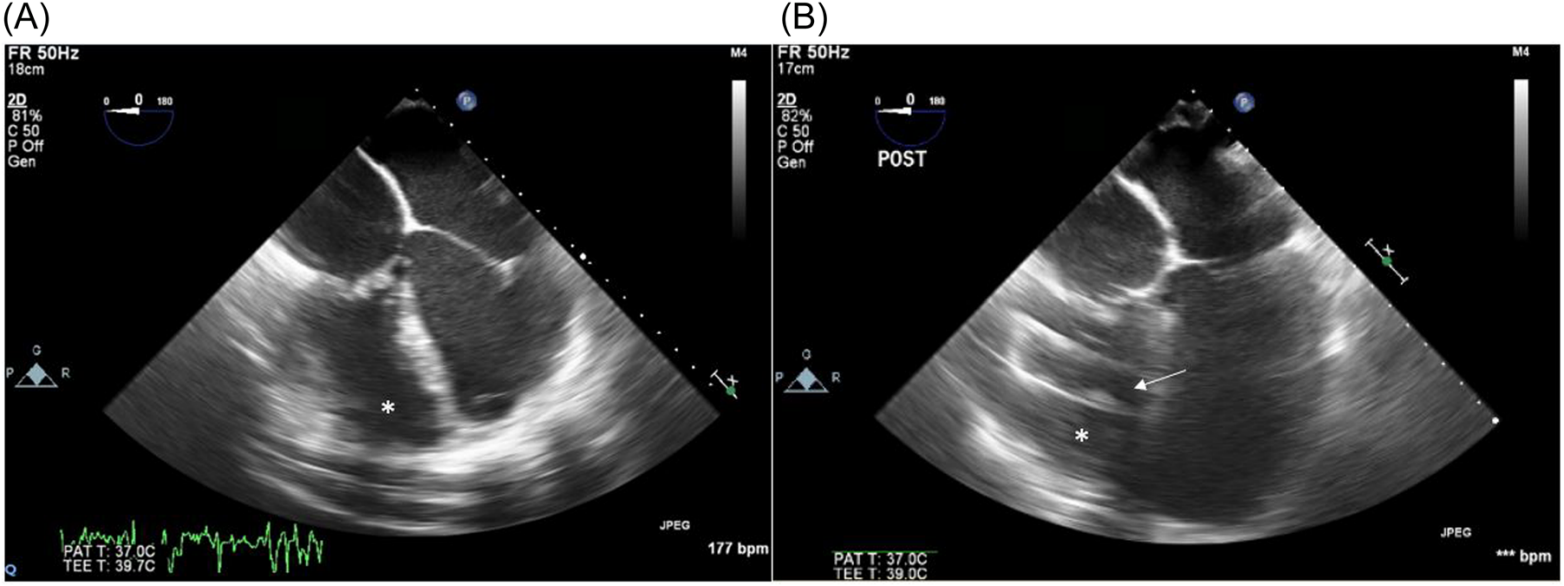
TEE images. (A) Preoperative four‐chamber view. *indicates the RV. (B) Postoperative four‐chamber view. The white arrow indicates the inflow cannula directed toward the right ventricular outflow tract. RV, right ventricle; TEE, transesophageal echocardiogram.
